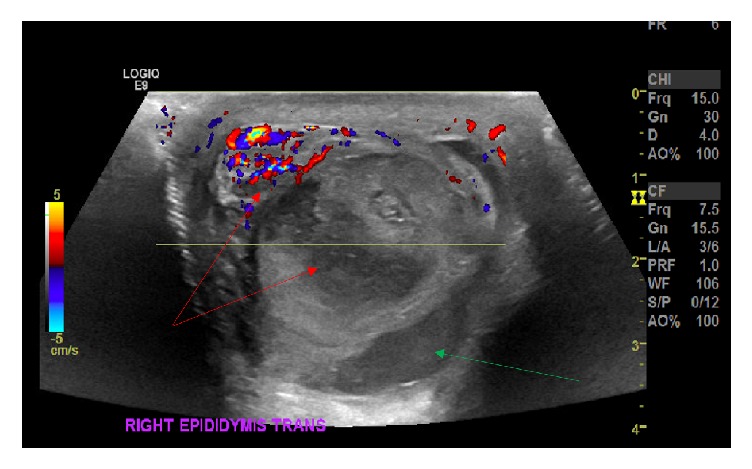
Doppler ultrasound performed upon patient presentation demonstrating heterogeneous echotexture in both the testicle and the epididymis signifying ischemia and inflammation in the testicle and necrosis in the epididymis (red arrows). Only peripheral blood flow to the testicle is present while blood flow to the epididymis is maintained. Additionally, a significant hematoma is visualized on the anterior aspect of the testicle (green arrow).
